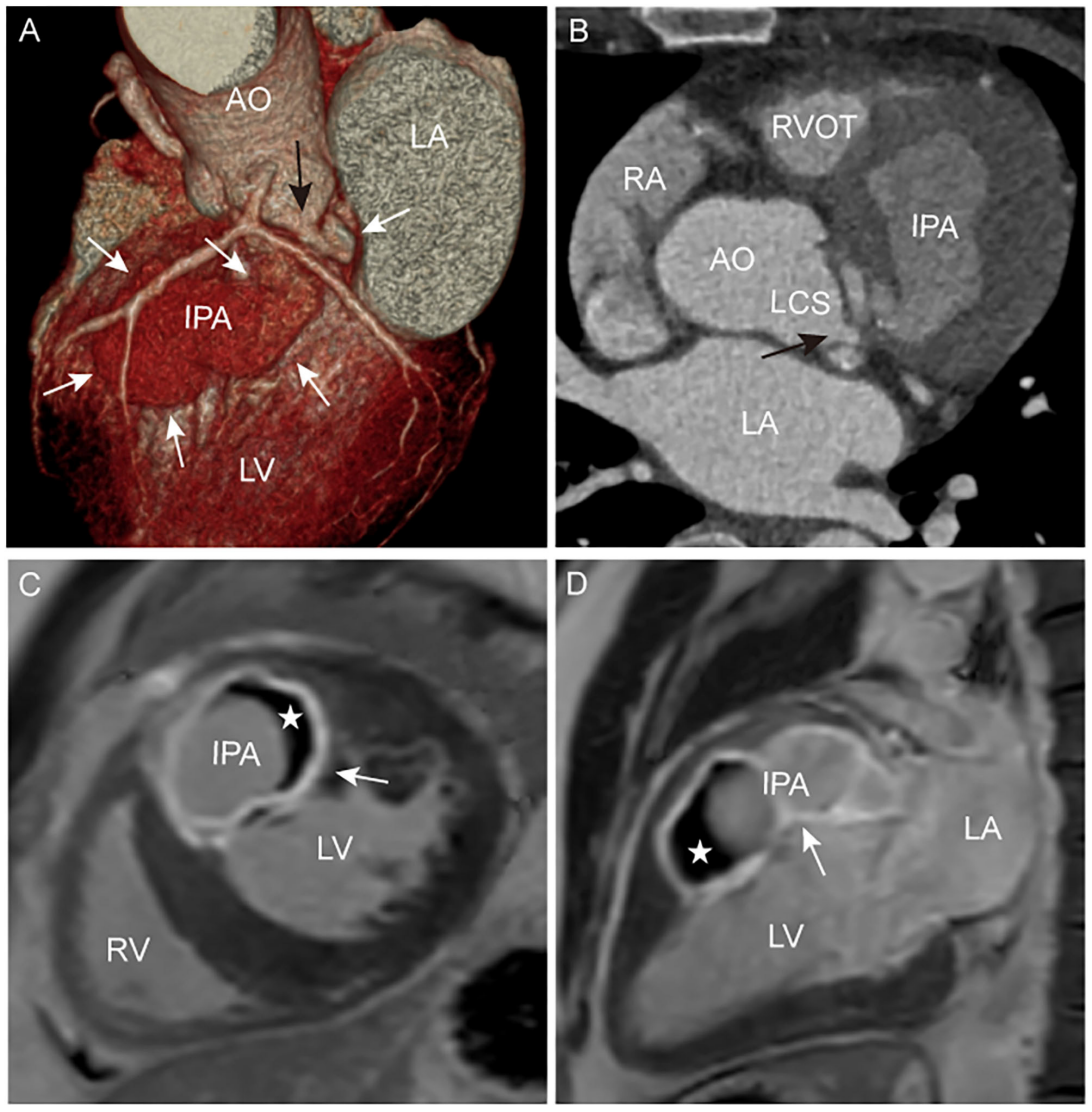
(A,B) CT angiogram images showing the anatomical profile of IPA (white arrow) and perforation (black arrow). (C,D) CMR LGE images showing the high signal intensity of the IPA with a distal low signal intensity (✰), and a linear high signal intensity (white arrow) of the IPA wall and partitions. LCS, left coronary sinus.
